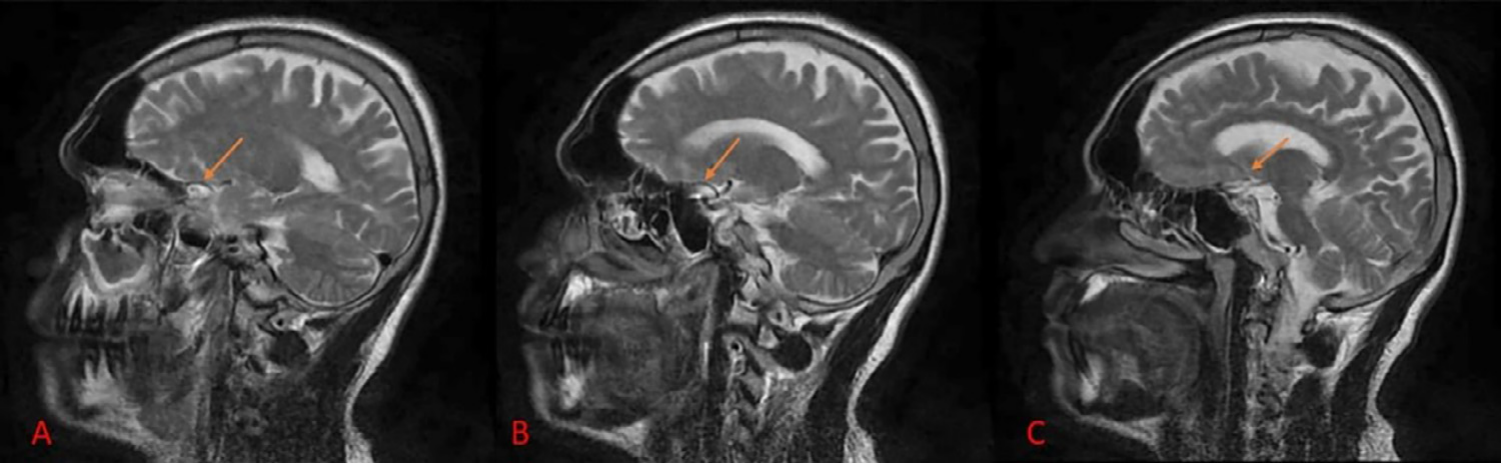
MRI brain sagittal image T2 weighted imaging (A-C) showing an intra-diploic lesion measuring approximately 14 × 7 mm adjacent to the left cavernous sinus, which appears isointense to CSF.
